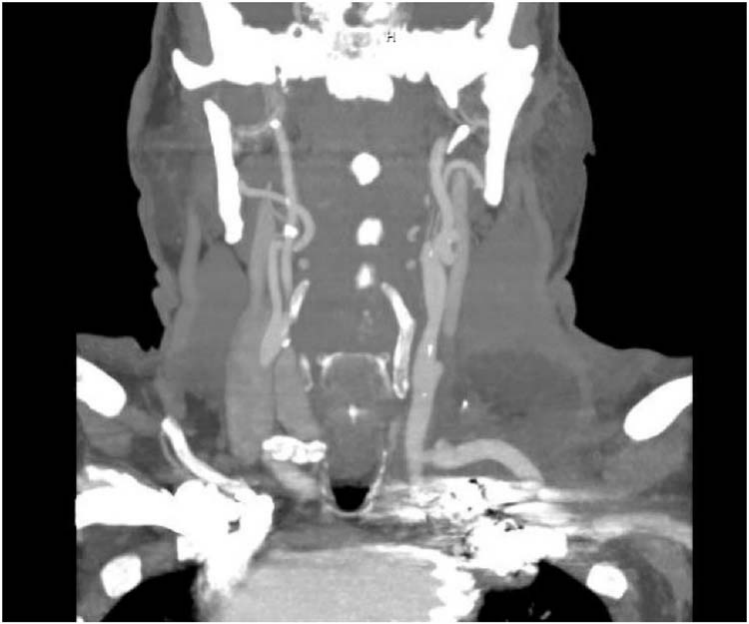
CT angiogram showing patency of the left carotid to subclavian artery bypass graft with vertebral artery implantation and showing coil embolization of the proximal left SAA.
